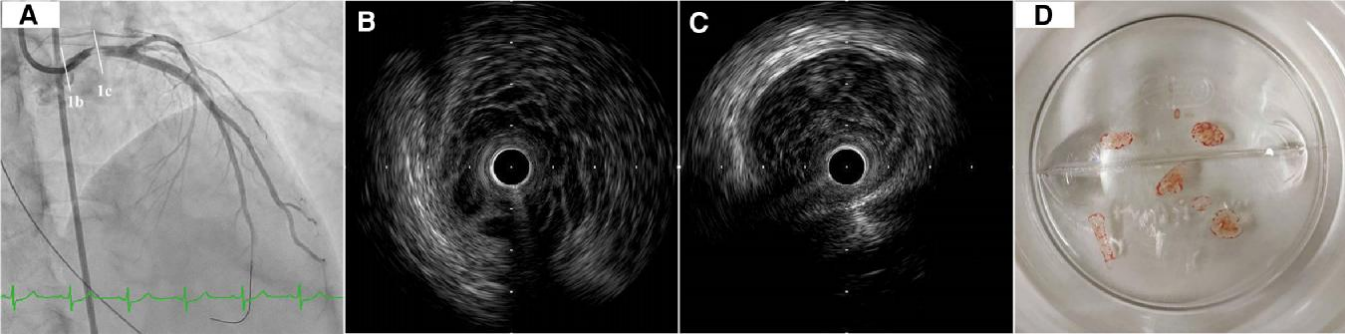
Coronary angiography, intravascular ultrasound (IVUS), and aspirated intracoronary mass findings. (*A*) Emergency coronary angiography demonstrating a mass occupying the left main coronary artery with delayed contrast filling. (*B*) IVUS demonstrating persistent protrusion of the mass from the left main trunk into the aortic root (line 1B). (*C*) IVUS demonstrating a heterogeneous, mulberry-like mass completely occluding the distal left main trunk (line 1C). (*D*) Materials aspirated using a suction catheter during thrombectomy. The fragments appeared colourless, transparent, and gelatinous.
